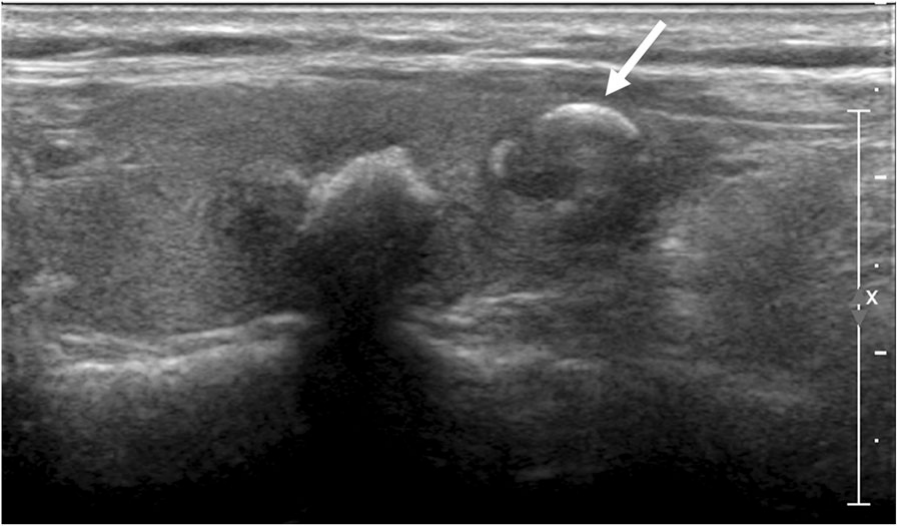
Echogenic foci associated with malignancy. 70-year-old female with thyroid nodule. Ultrasound image shows a 1.0 cm hypoechoic, solid nodule with peripheral curvilinear echogenic foci (Type 5, arrow). Biopsy result was papillary carcinoma and was confirmed at surgery
